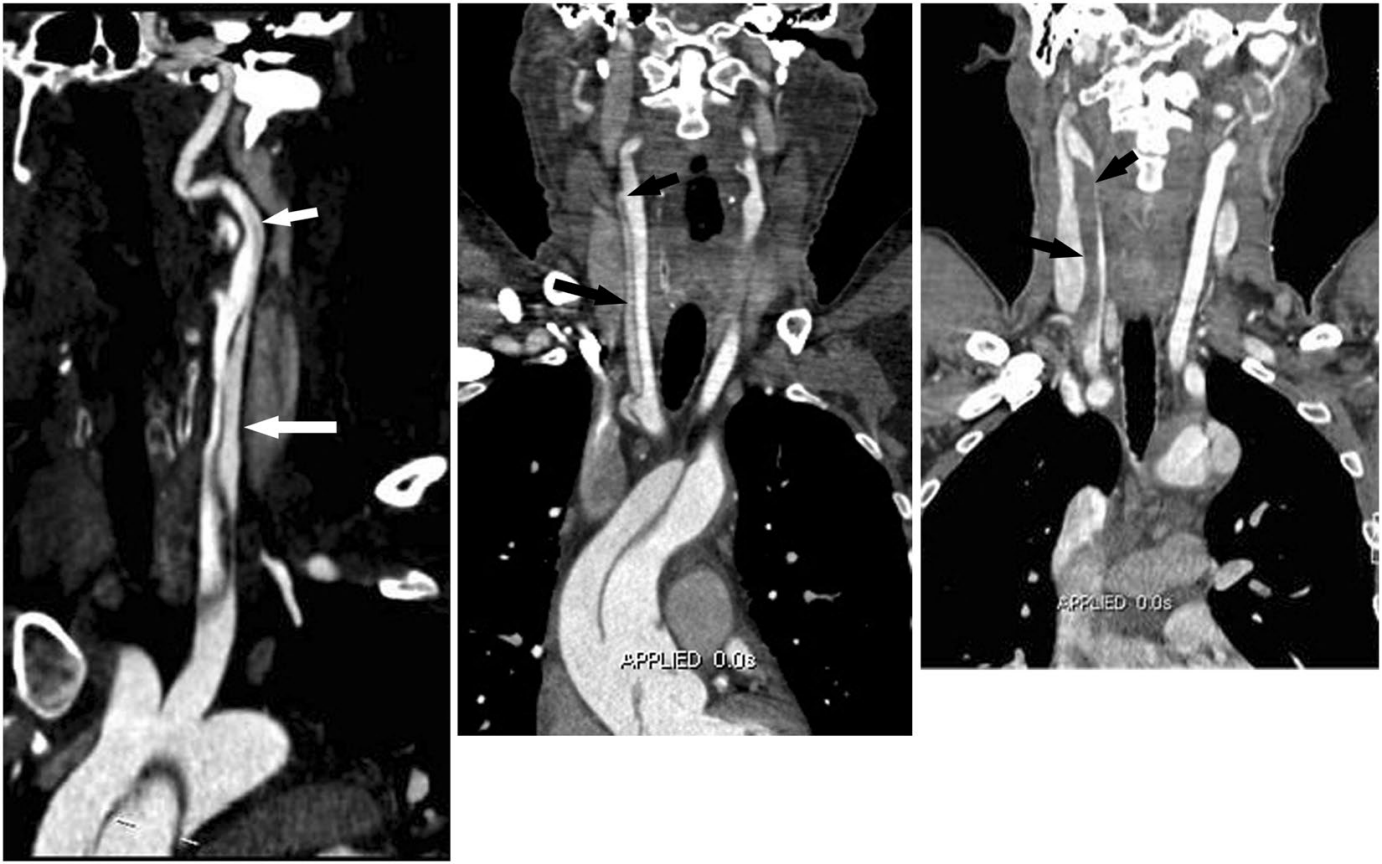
Significant Detailed Findings of CCA Dissection for Postoperative TND. (a) CPR image showing aortic dissection involving the left CCA (long arrow) and proximal ICA (short arrow). Patients with a CCA dissection longer in length involving the entire CCA and proximal ICA exhibit a significantly higher incidence of postoperative TND than those with CCA dissection involving either the CCA alone or the proximal CCA. (b) Coronal MPR image showing aortic dissection involving the right CCA (long arrow) with a re-entry tear (short arrow) in the distal CCA. Note that the density of the false lumen is lower than that of the true lumen. This finding indicates a higher risk of the thrombus in the low-enhancement false lumen extending to the cerebrum via the re-entry point. (c) Coronal MPR image showing aortic dissection extending into the right CCA. CCA dissection without re-entry in the distal CCA and thrombosis in the false lumen (arrow). The severely narrowed true lumen (arrow head) resulting from the compression of a haematoma in the false lumen implies probable cerebral hypoperfusion due to a decreased blood supply from the true lumen of the CCA. CTA = CT angiography; CPR = curved multiplanar reformation; CCA = common carotid artery; MPR = multiplanar reformation.
